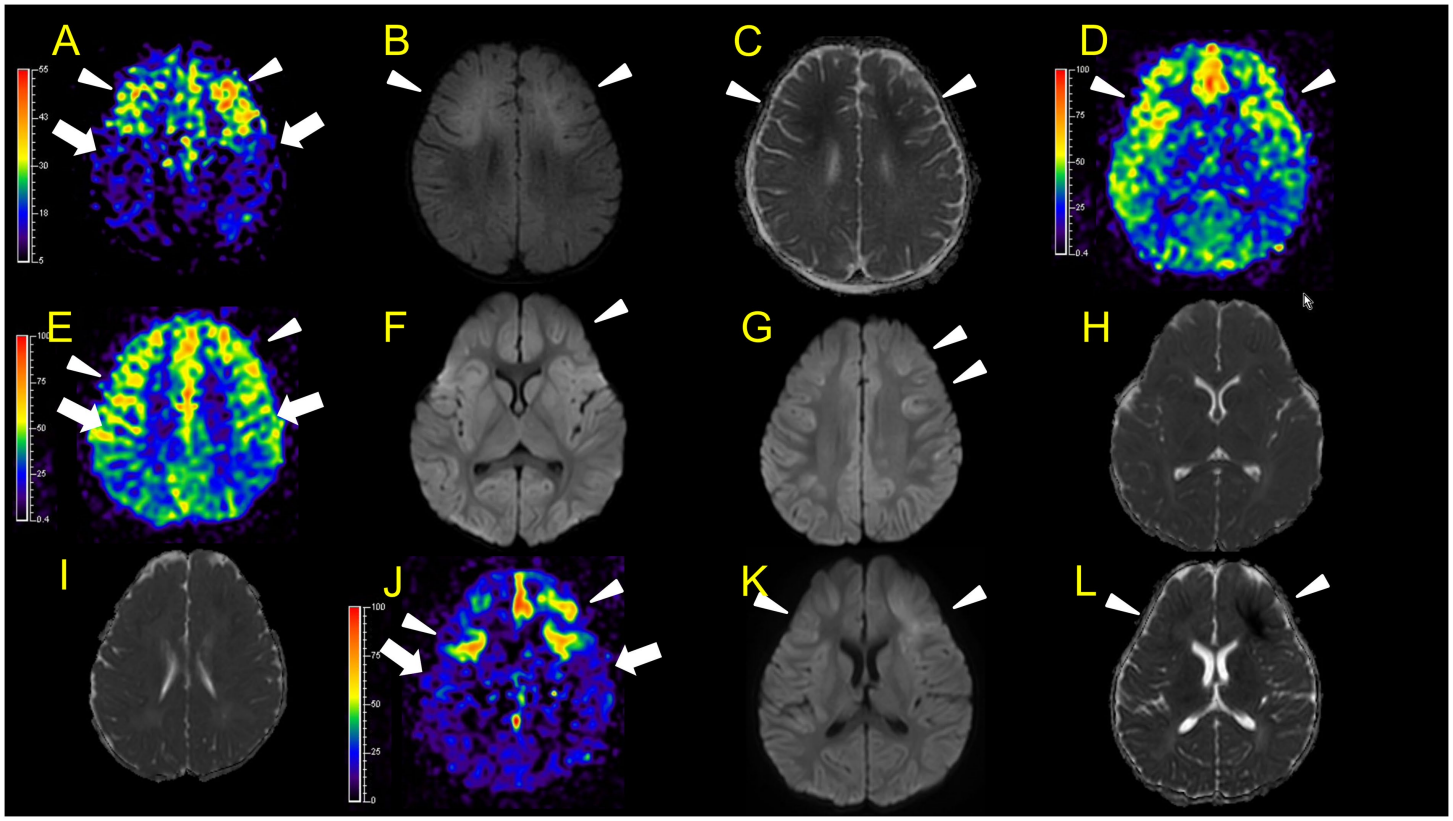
Neuroimaging findings. Color bars for ASL indicate signal intensities, not absolute perfusion values. Patient 1 (A–C): ASL (post-labelling delay 1800 ms), DWI, ADC on day 7. Hyperperfusion areas (A: arrowheads) in bilateral frontal regions coincide with lesions in DWI and ADC (B,C: arrowheads). Patient 2 (D–L): D–I, ASL (D,E, post-labelling delay 2000 ms), DWI (F,G), ADC (H,I) at 31 h after ESs (E,G,I are higher slices of D,F,H). There are hyperperfusion areas in the bilateral prefrontal regions (bilateral middle frontal lobes and left medial prefrontal cortex; D: arrowheads) compared to perirolandic regions (arrows). There are slight abnormal changes in DWI (F, G: arrowheads) in the left frontal region, which were not accompanied by apparent diffusion coefficient abnormalities (H,I). (J–L) ASL (post-labelling delay 2000 ms), DWI, and ADC at 82 h after ESs. ASL (J: arrowheads) exhibited more prominent hyperperfusion areas than the first ASL image compared to perirolandic regions (J: arrows). On DWI and ADC, BTA appeared on bilateral frontal regions (K,L, arrowheads).
